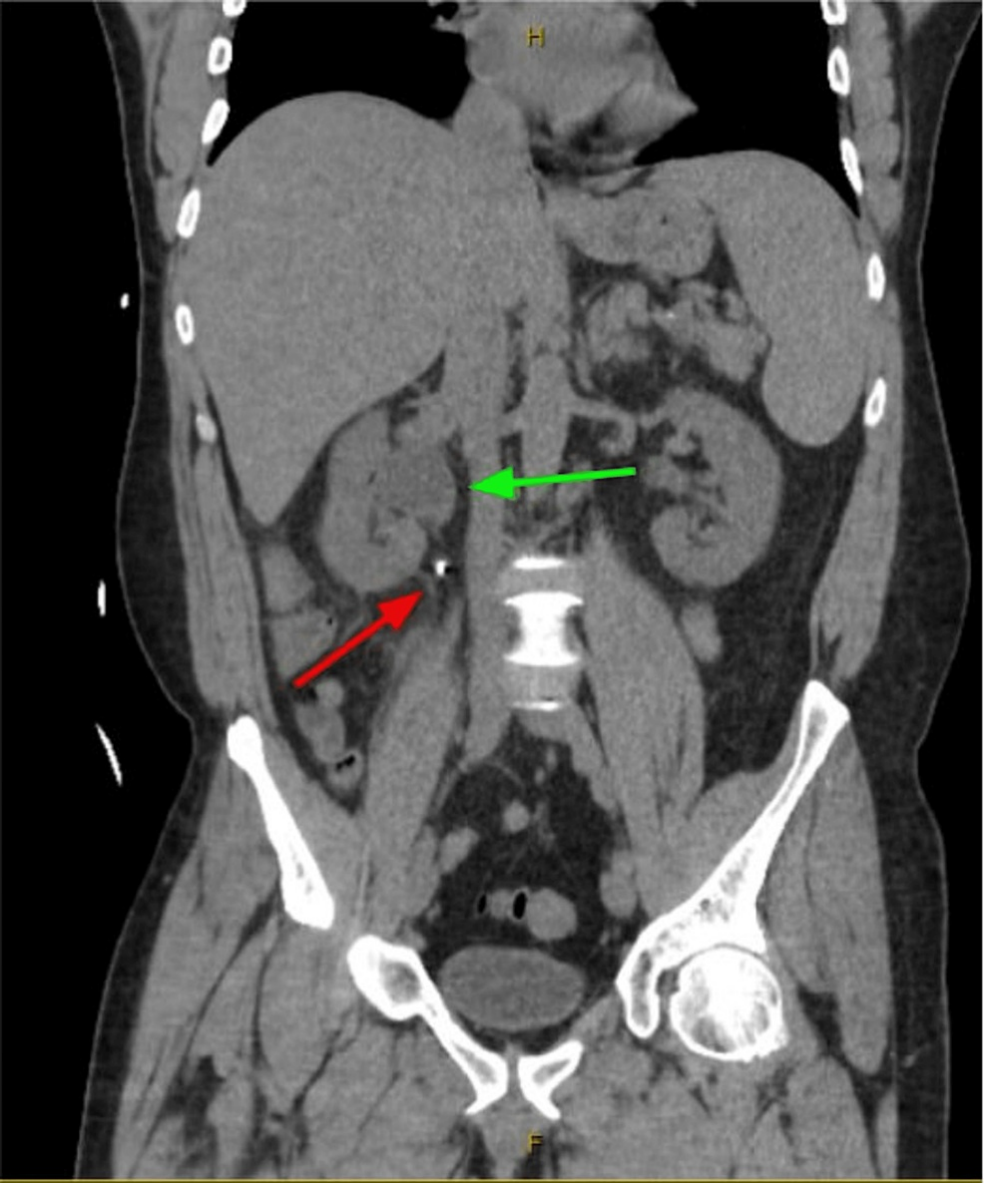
CT abdomen showing 7mm right ureteral stone (red arrow) with hydronephrosis (green arrow)
